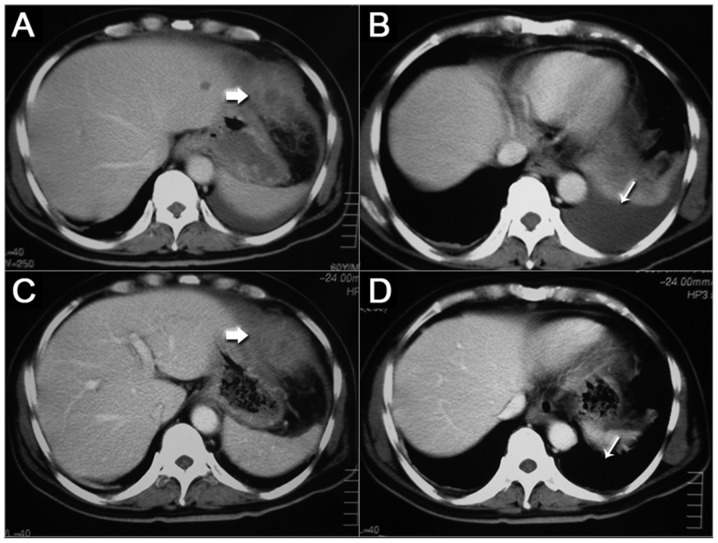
Computed tomography (CT) results of the patient prior to admission to The First Affiliated Hospital of Sun Yat-Sen University (Guangzhou, Guangdong, China). (A and B) Horizontal CT scan prior to a percutaneous thoracic drainage procedure. An extremely large mass, 20×15×8 cm in size, was located in the splenic curve of the colon, and a left-sided pleural effusion can be distinctly observed. (C and D) Horizontal CT scan following a percutaneous thoracic drainage procedure. Wide arrows indicate the mass under the diaphragm, whereas thin arrows mark the regions of pleural effusion. The resolved pleural effusion (D) reoccurred shortly after admission to The First Affiliated Hospital of Sun Yat-Sen University.
